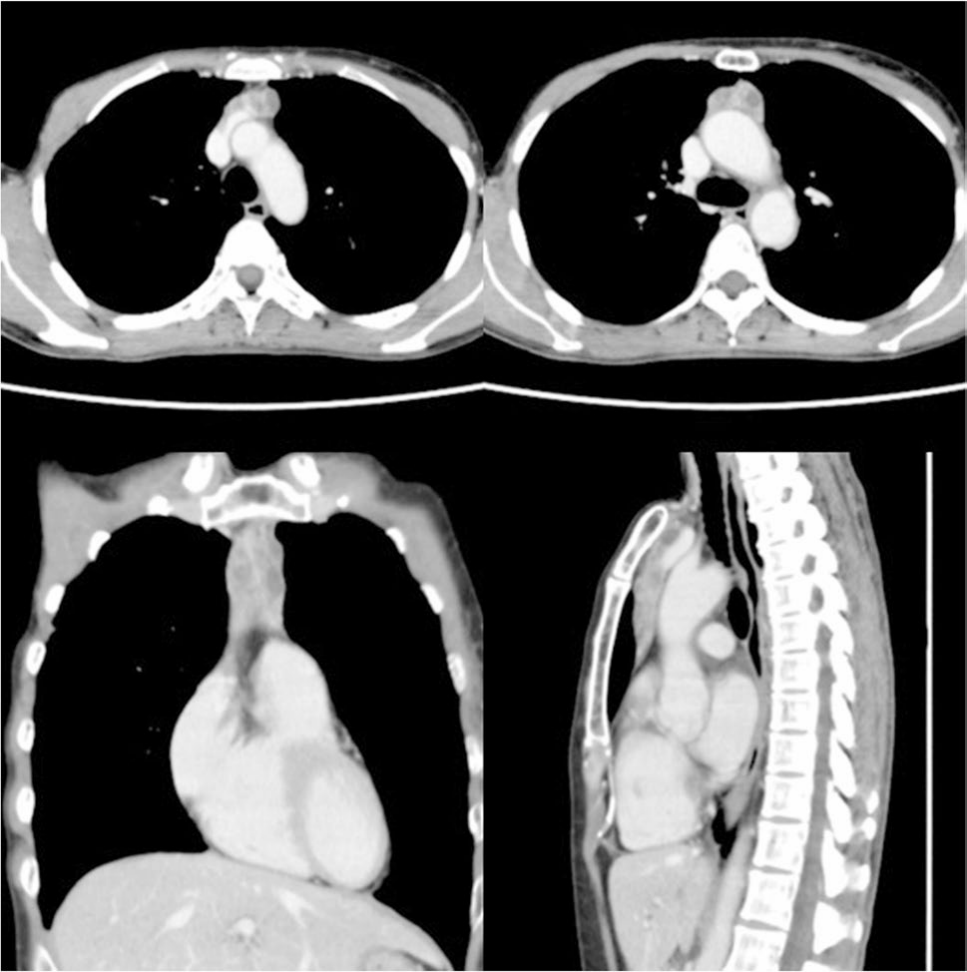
CT scan revealed multiple nodules containing a mixture of solid and cystic components in the thymus, indicating possible invasion into the left brachiocephalic vein
